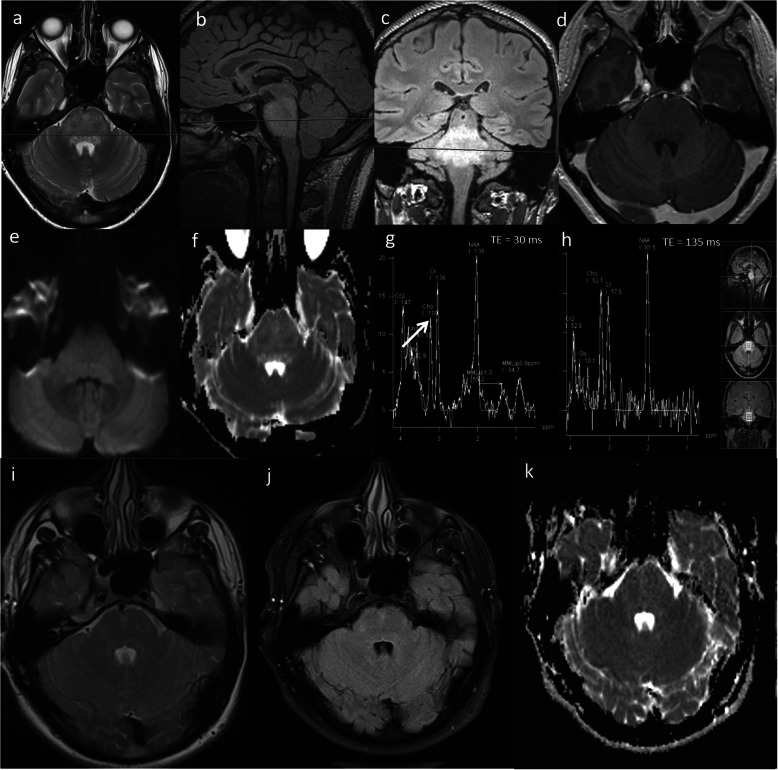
Bickerstaff brainstem encephalitis. Conventional MRI Findings: (a) Axial T2W, (b, c) sagittal and coronal FLAIR and (d) axial post-contrast T1W sequences, show a diffuse high signal lesion in the pons with no enhancement post-contrast. Multiparametric MRI: e, f DWI shows high ADC throughout the lesion (> 1000 × 10^−6^ mm^2^ s^−1^). g, h MRS shows normal mI/Cr, normal Cho/Cr (arrow) and normal NAA/Cr ratios and minimally increased glutamine and glutamate peaks (2.3 and 2.4 ppm). PWI (not shown) had low rCBV compared to normal-appearing white matter. The lack of enhancement, low rCBV, high ADC and normal choline exclude glioma. These multiparametric MRI features in conjunction with an acute presentation favour an inflammatory lesion. Two-month follow-up imaging: (i) axial T2W, (j) FLAIR and (k) ADC sequences show lesion regression and normalisation of diffusion. In this case, CSF analysis revealed antiganglioside antibodies consistent with a diagnosis of Bickerstaff brainstem encephalitis
